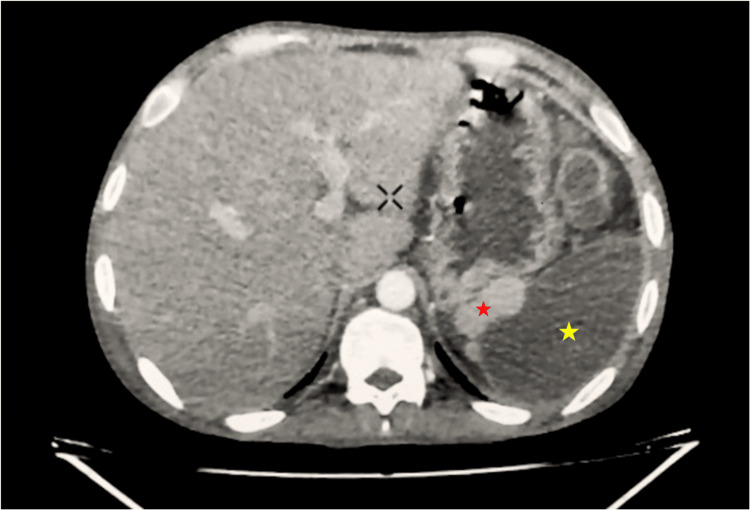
Contrast-enhanced CT abdomen (axial view) demonstrating markedly heterogeneous hypoattenuation of the splenic parenchyma with near-complete absence of contrast enhancement, consistent with extensive splenic devascularization The spleen is largely replaced by low-attenuation areas with internal heterogeneity, indicative of advanced liquefactive necrosis in the setting of chronic post-traumatic injury. The yellow star denotes regions of complete liquefaction (near fluid attenuation) within the spleen. The red star highlights adjacent hyperdense foci, likely representing residual vascular structures or contrast opacification near the splenic hilum.
